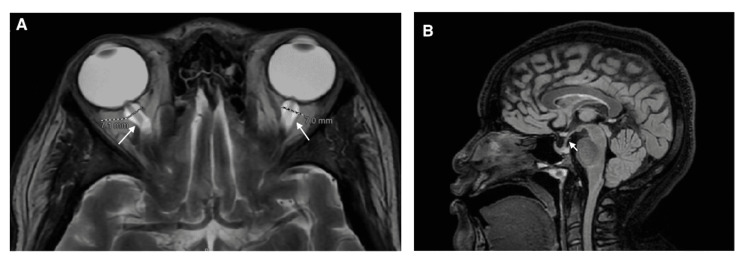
Brain MRI showing signs of an increased intracranial pressure without space-occupying lesions. (a) Axial T2-weighted imaging (T2WI) showing optic nerve sheath edema, evidenced by an increase in optic nerve sheath diameter (OSND) of > 6 mm bilaterally (normal OSND between 4.8 and 6 mm). (b) Sagittal fluid-attenuated inversion recovery (FLAIR) sequence showing empty sella turcica with infundibulum sign.
